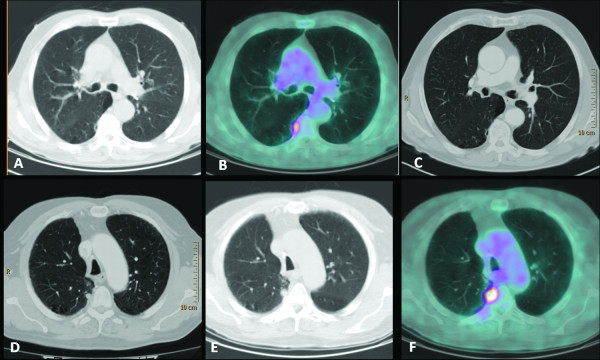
The undetectable nodule. ^18^ F-FDG PET-CT (June 2010): CT (a) and fused (b) axial images. Focal ^18^ F-FDG uptake is evident in the subpleural parenchyma of the apical segment of the right lower lobe. (c) Contrast-enhanced CT axial image (June 2010): no significant alterations in the site of ^18^F -FDG uptake. (d) Contrast-enhanced CT axial image (January 2011): a pulmonary nodule of 1 cm in the apical segment of the right lower lobe, corresponding to the site of ^18^F -FDG uptake is revealed. ^18^ F-FDG PET-CT (April 2011): CT (e) and fused (f) axial images. Clear evidence in the apical segment of the right lower lobe of a pulmonary nodule characterized by intense metabolic activity.
